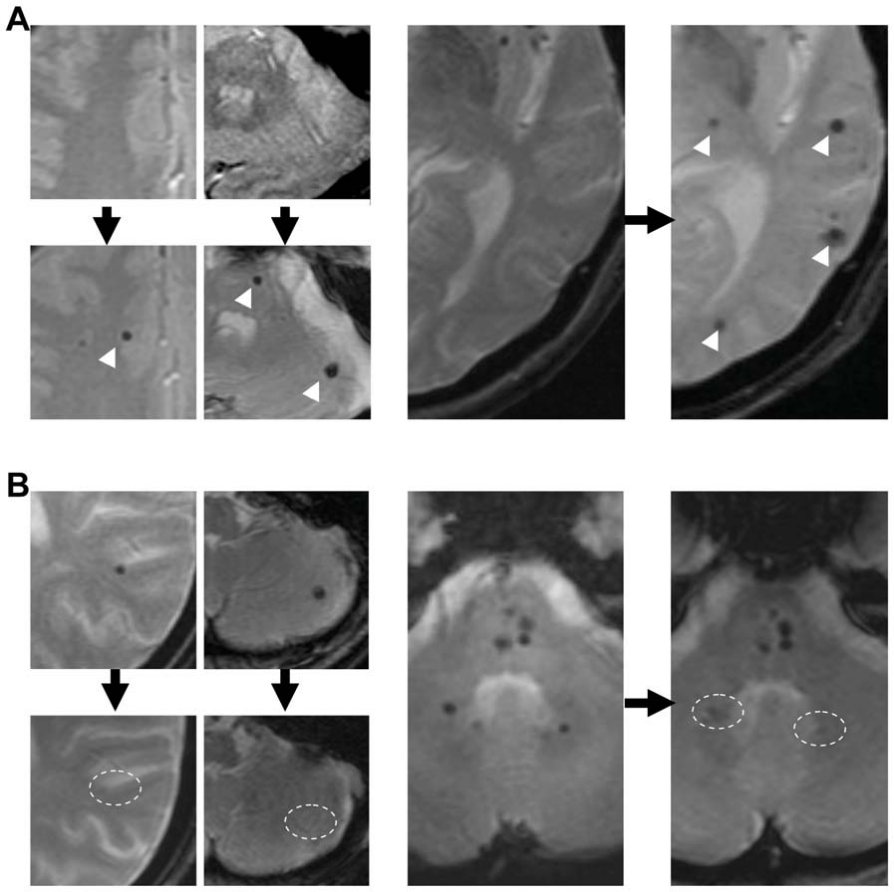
Dynamic changes of MBs over a long-term MRI follow-up. Some new MBs appeared (A), and some MBs disappeared in the follow-up MRI (B). Black arrows represent the MRI follow-up, while white arrow heads indicate new MBs, and dotted circles identify the location of those MBs which had disappeared.
